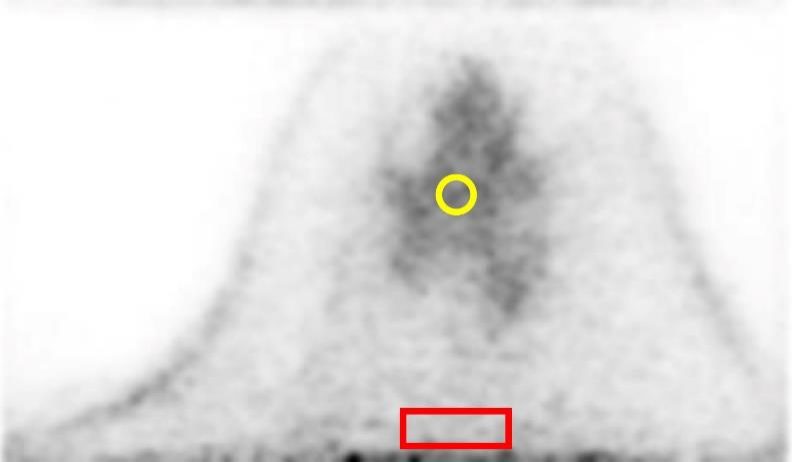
Placement of regions of interest (ROI) in the measurement of dbPET images. The SUV_mean_ and CV of the background fibroglandular tissue were obtained from five 2D-ROIs with a diameter of 8 mm (yellow) and the CV of the background fibroglandular tissue or fat tissue at the edge of the FOV were obtained from five 2D-ROIs of 10×30 pixel rectangles placed at 5 pixels from the FOV edge (red). Each ROI was placed in 5 different slices, which are at least 5 slices apart from each other to include as wide range of background fibroglandular tissue as possible
